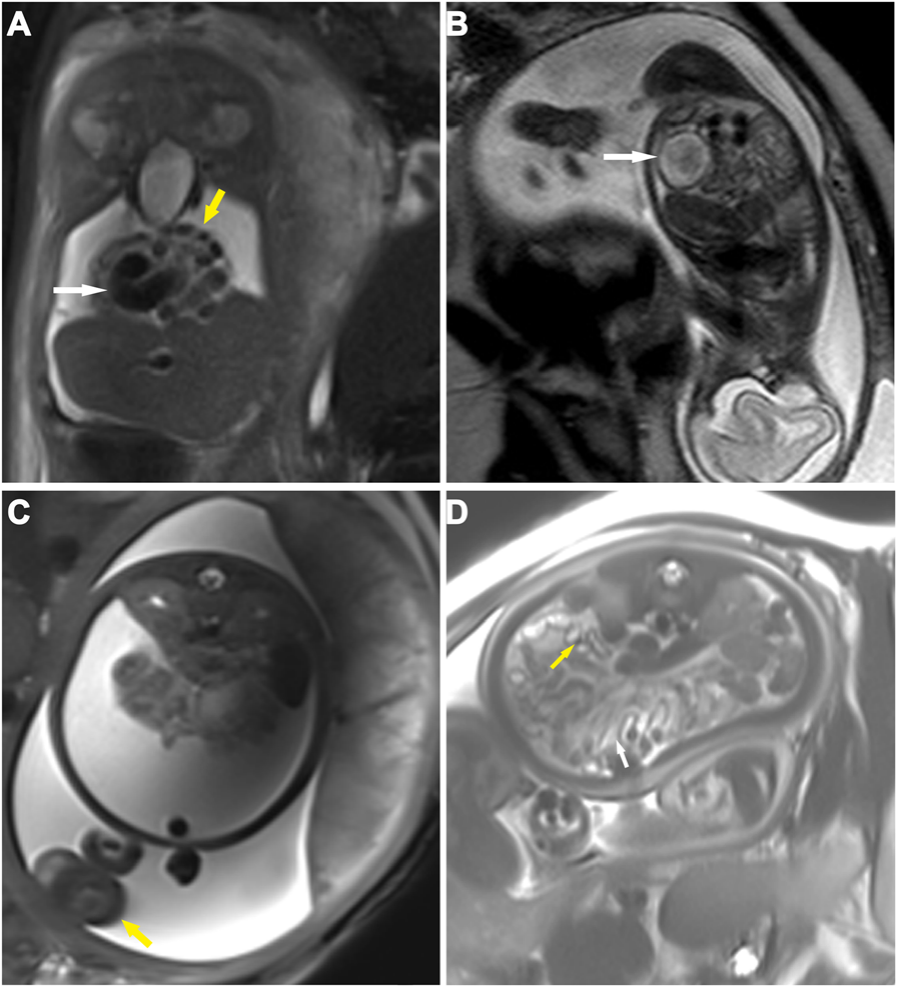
Fetal MRI findings of meconium peritonitis. a A coronal 3.0-T fat-suppression T2-weighted image (HASTE, TR = 15,000 ms, TE = 91 ms) reveals a moderate amount of ascites (a low-intensity signal) in the peritoneal cavity, compacted bowel loops (yellow arrow) and dilated bowels (white arrow) in a 30-week-old fetus with meconium peritonitis (MP). b A sagittal 1.5-T fetal T2-weighted image (TR = 15,000 ms, TE = 120 ms) shows mild ascites among bowel loops and a cyst (a 3.2 × 2.4 × 3.2 cm round foci with mix signal inside, white arrow) in a 24-week-old fetus with MP. c A transverse 3.0-T HASTE-FS image (TR = 15,000 ms, TE = 91 ms) indicates a high mount of ascites, gathered bowel loops (white arrow) and enlargement of the left scrotum (yellow arrow) in a 37-week-old fetus with MP. d A transverse 3.0-T fat-suppression T2-weighted image (HASTE, TR = 15,000 ms, TE = 91 ms) indicates gathered bowel loops (white arrow) and calcification spots (multiple small focuses or small nodules with low T2-weighted imaging signal, yellow arrow) in a 38-week-old fetus with MP
